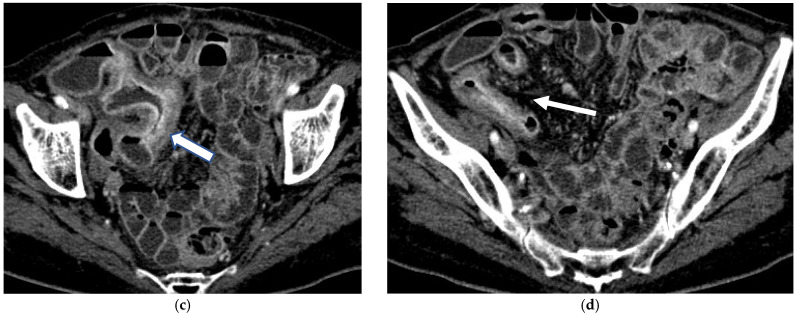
(a,b) Coronal CT scan in the venous phase after administration of negative oral contrast medium (PEG-solution) showing thickened ileal loops (arrows) in an 85-year-old female patient with Crohn’s disease. (c,d) axial CT scan in the venous phase after administration of negative oral contrast medium (PEG-solution) showing thickened ileal loop (arrows) in an 85-year-old female patient with Crohn’s disease.
